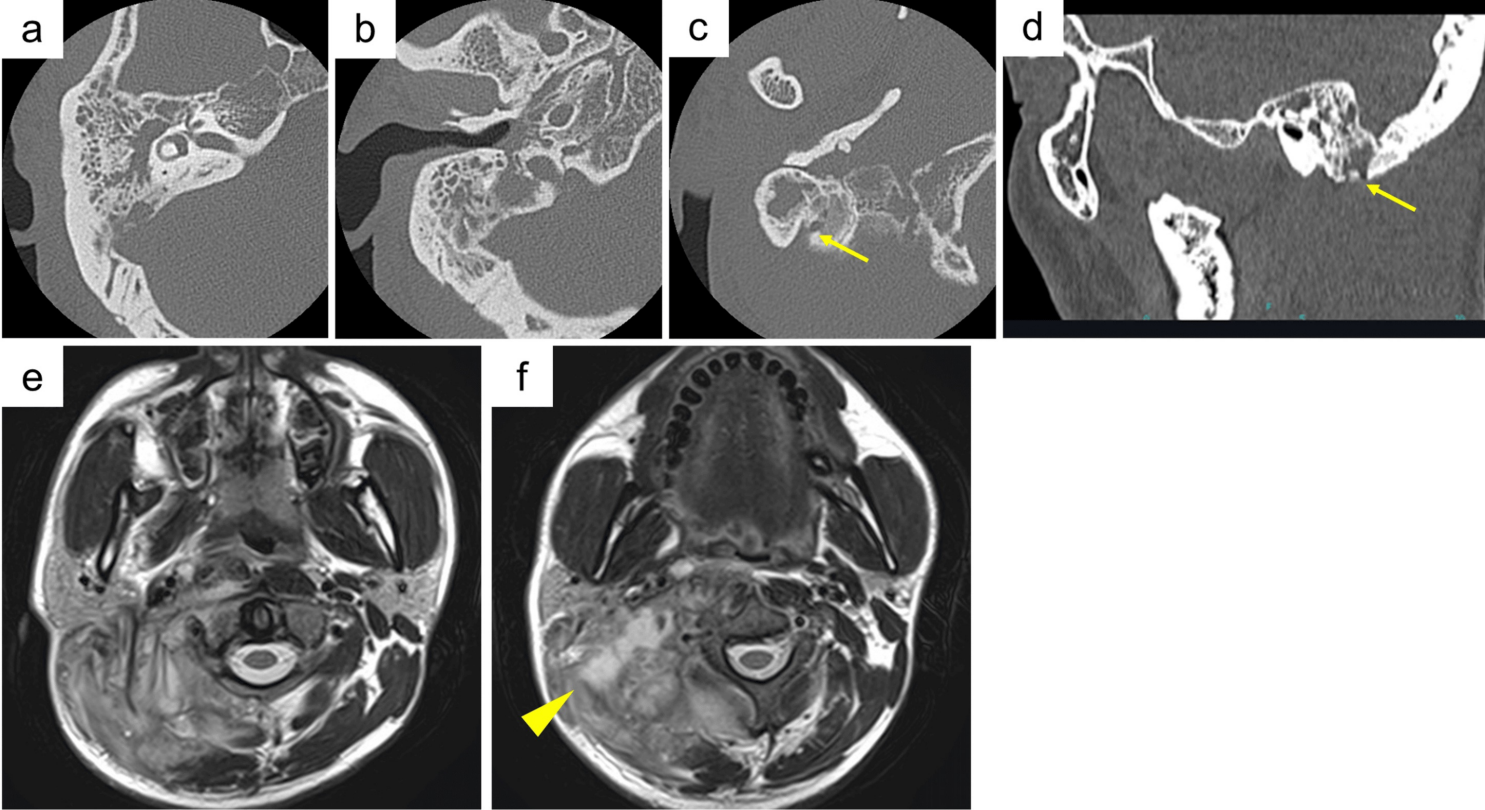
Imaging findings on presentation. (a) Axial target CT of the temporal bone shows complete opacification of the left mastoid air cells.
